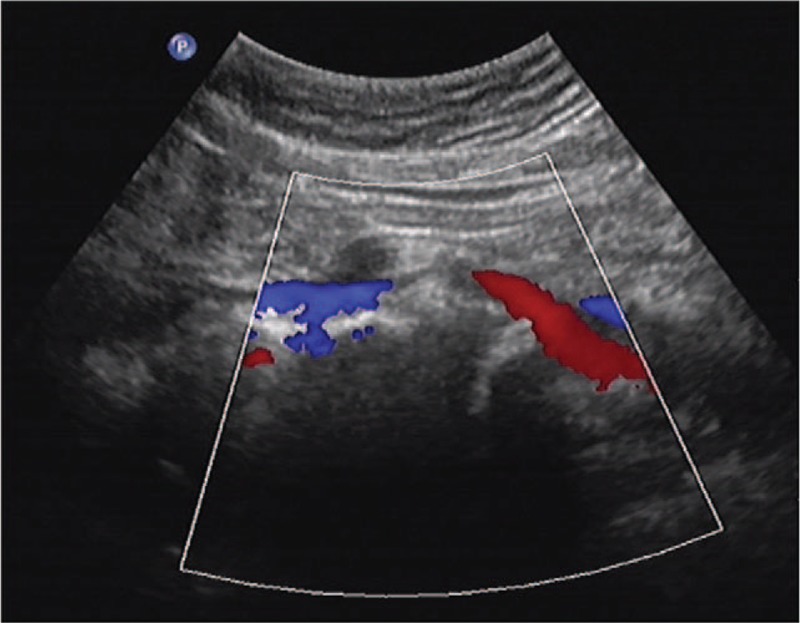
Postoperative abdomen Doppler shows that as the stenosis of inferior vena cava was relieved, the venous patency increased to 70%.
